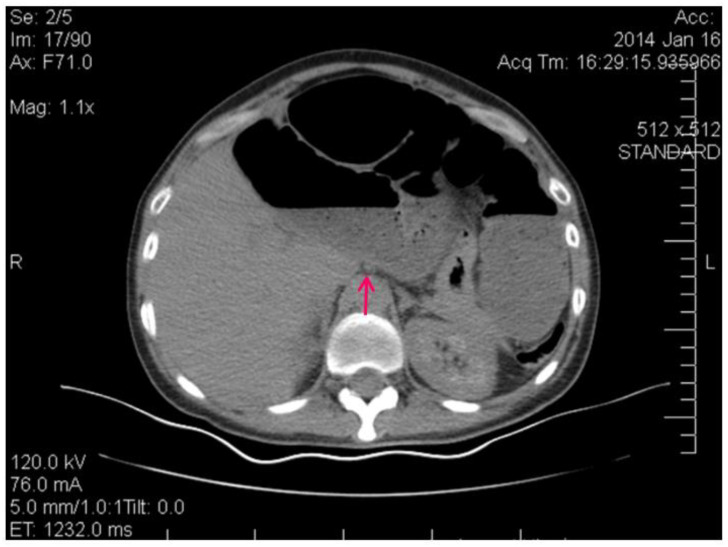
CT image of the “Whirlpool sign”— spiralling appearance of the mesenteric vessels (red arrow) suggestive of a small bowel volvulus.
